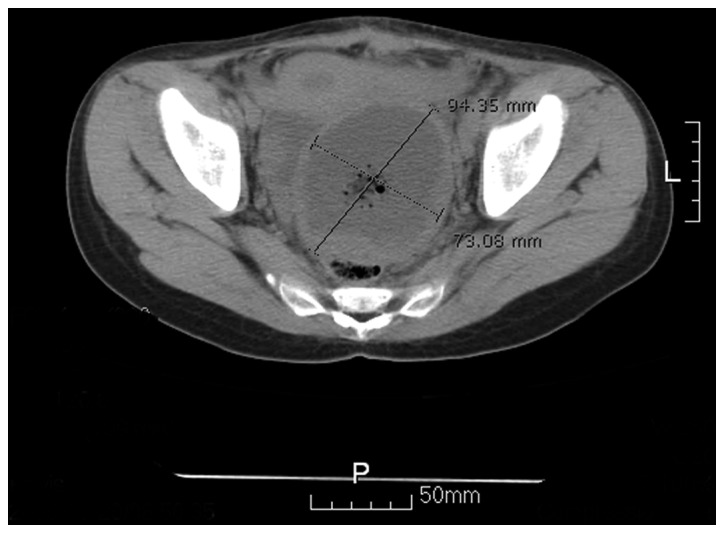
Computed tomography scan of the pelvic mass (size, 9.4×7.3 cm).
